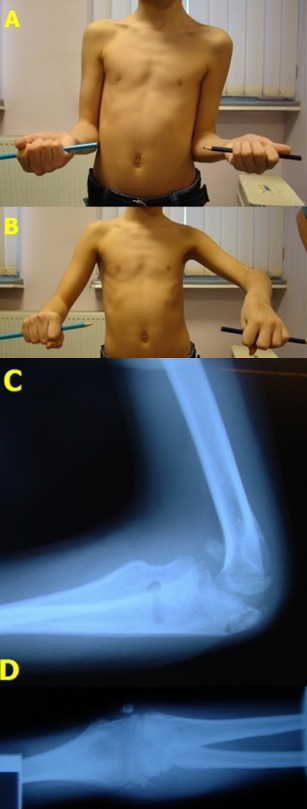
Clinical and radiological 2 months follow-up. A. Normal supination. B. 20 degrees limited left antebrachial pronation. C. Lateral X-rays showing the limiting of radial head relaxation. D. Normal positioning of the radial head.
